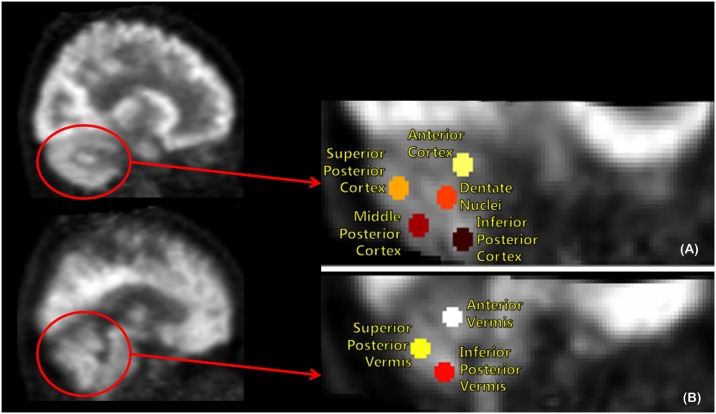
Volume of Interest (VOI) template on the cerebellum of a normal sagittal ^18^F-FDG PET image includes 13 VOIs in bilateral anterior cortex, superior/mid/inferior posterior cortex, dentate nuclei (A), anterior vermis, and superior/inferior posterior vermis (B).
